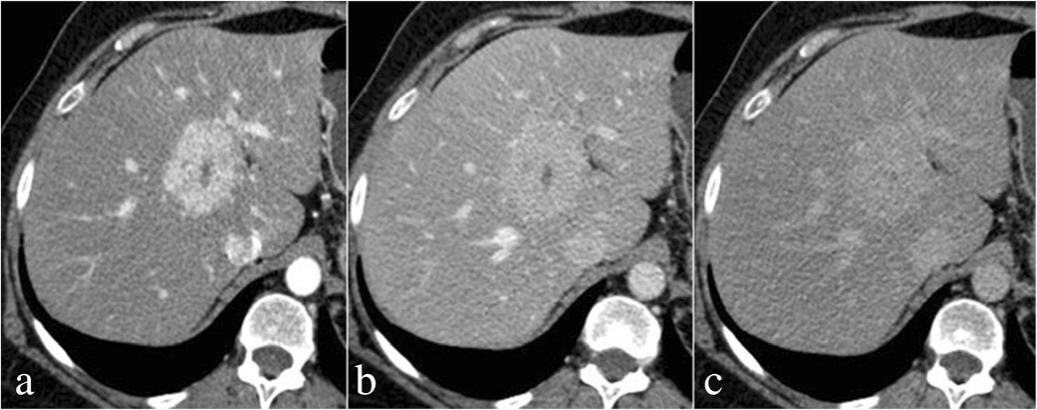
Triphasic 64-detector row computed tomography findings of focal nodular hyperplasia in a 53-year-old woman. Arterial phase contrast-enhanced computed tomography scan shows intense homogeneous enhancement with hypodense focal central scar (a); contrast-enhanced computed tomography scan during the portal venous phase shows lesion exhibiting rapid contrast material washout being slightly hypo-attenuated compared with surrounding liver (b); delayed phase contrast-enhanced computed tomography scan shows lesion as isodense and persistent enhancement of central scar (c).
